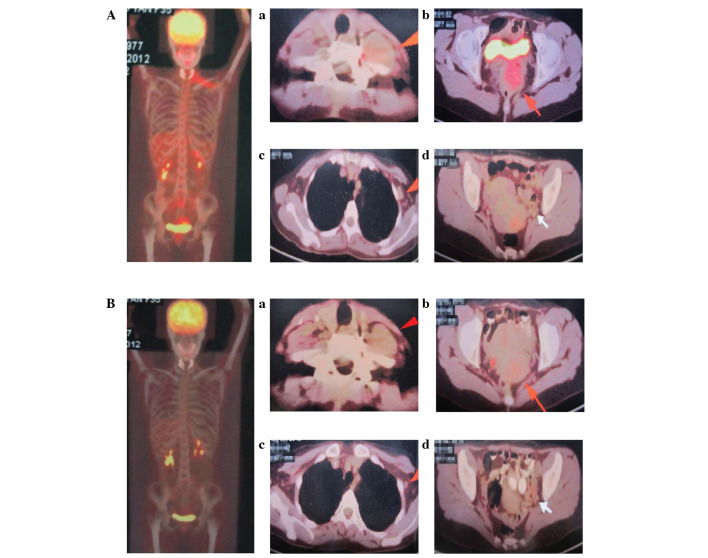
(A) FDG-PET showed hypermetabolic lesions (arrows) in the (a) left trapezius muscle, (b) cervix uteri, (c) lymphadenectasis of the left axillary fossa and (d) pelvic wall. (B) FDG-PET showed a decrease in FDG uptake following chemotherapy (a–d). FDG, fluorodeoxyglucose; PET, positron emission tomography.
